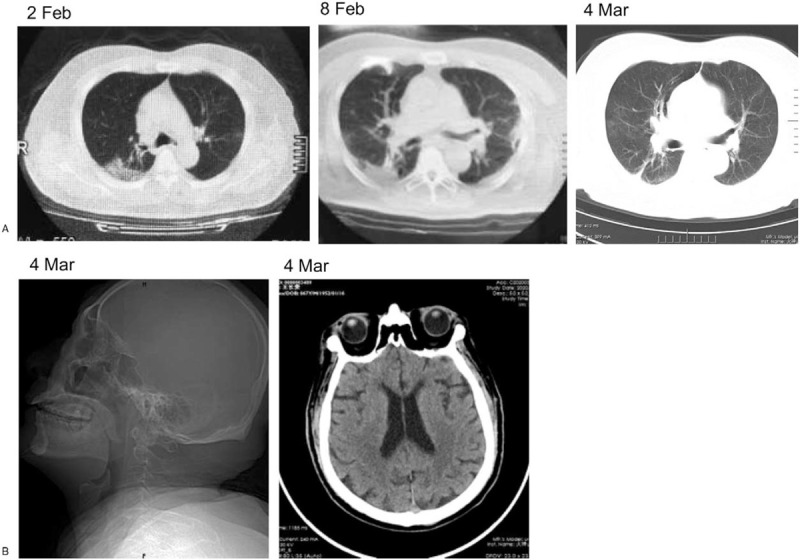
Craniocerebral and chest computed tomography (CT) of this patient. A. Chest CT changes in this patient: lesions were distinctly increased and enlarged on February 8 compared with those on February 2, and shadows and lesions were obviously decreased and ameliorated. B. Craniocerebral CT: lacunar lesions in the left basal ganglia region. CT = computed tomography.
